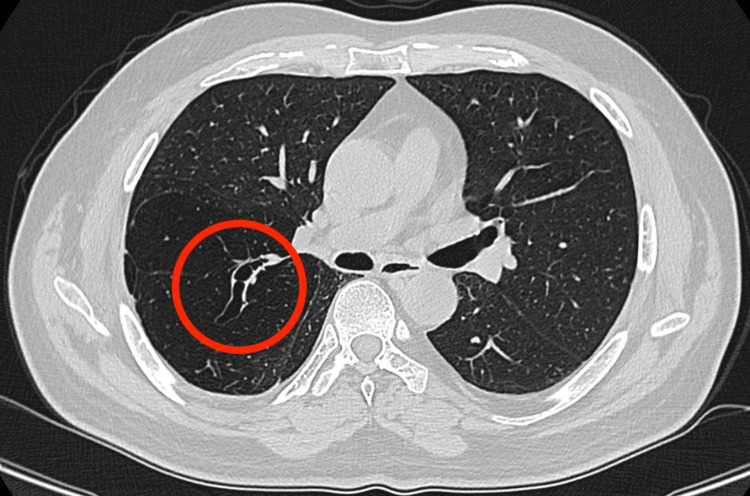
Bronchocele with mucoid impaction Axial chest CT image in lung window demonstrating a branching tubular opacity consistent with a bronchocele containing mucoid impaction within the affected subsegmental bronchus (red circle).
